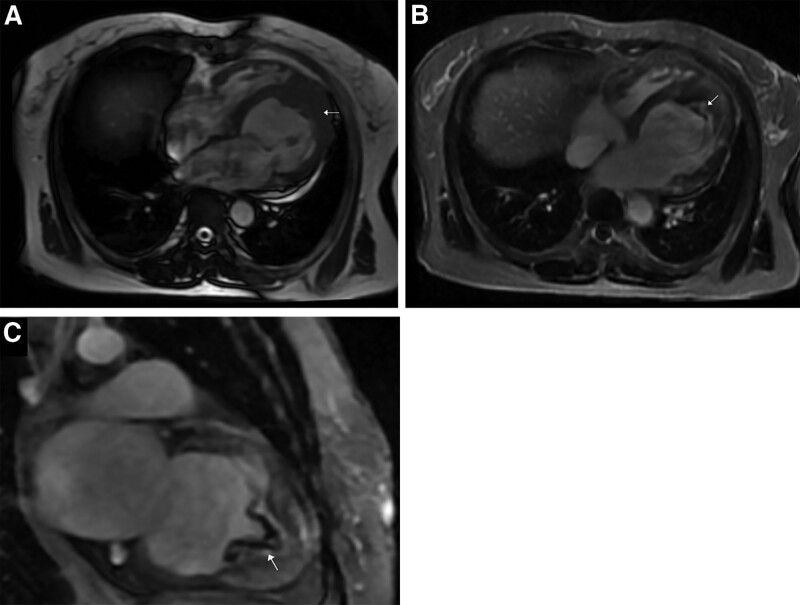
Cardiac magnetic resonance imaging showing apical hypertrophy (A, white arrow) and late gadolinium imaging indicating endomyocardial fibrosis of the left ventricle (B and C, typical “double V” sign, subendocardial enhancement with overlying thrombus).
